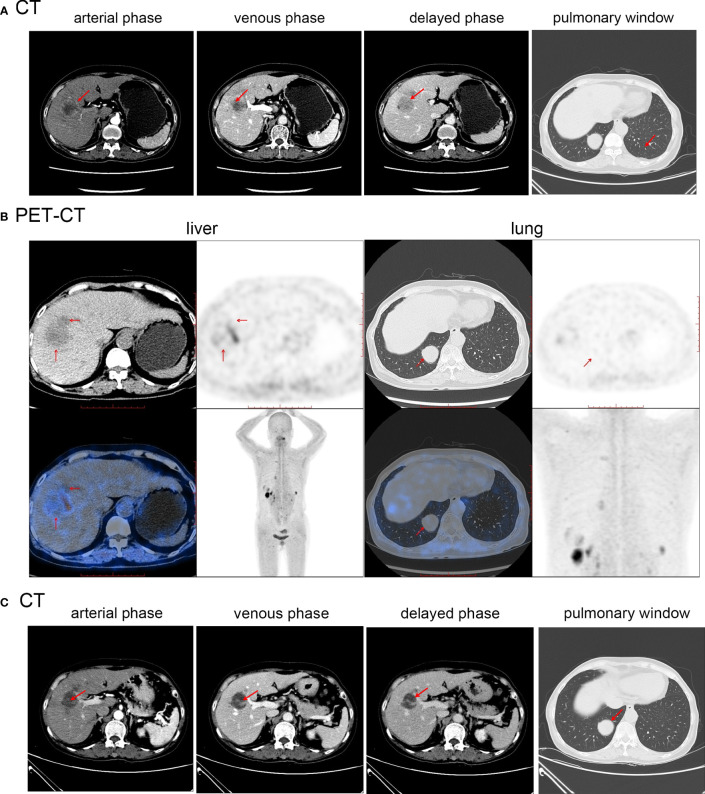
(A) Abdominal MR before first treatment of Case2. (B) Abdominal PET-CT before initial treatment of Case2. (C) Abdominal MR after first treatment of Case2. Red arrows indicate the location of the tumor.
